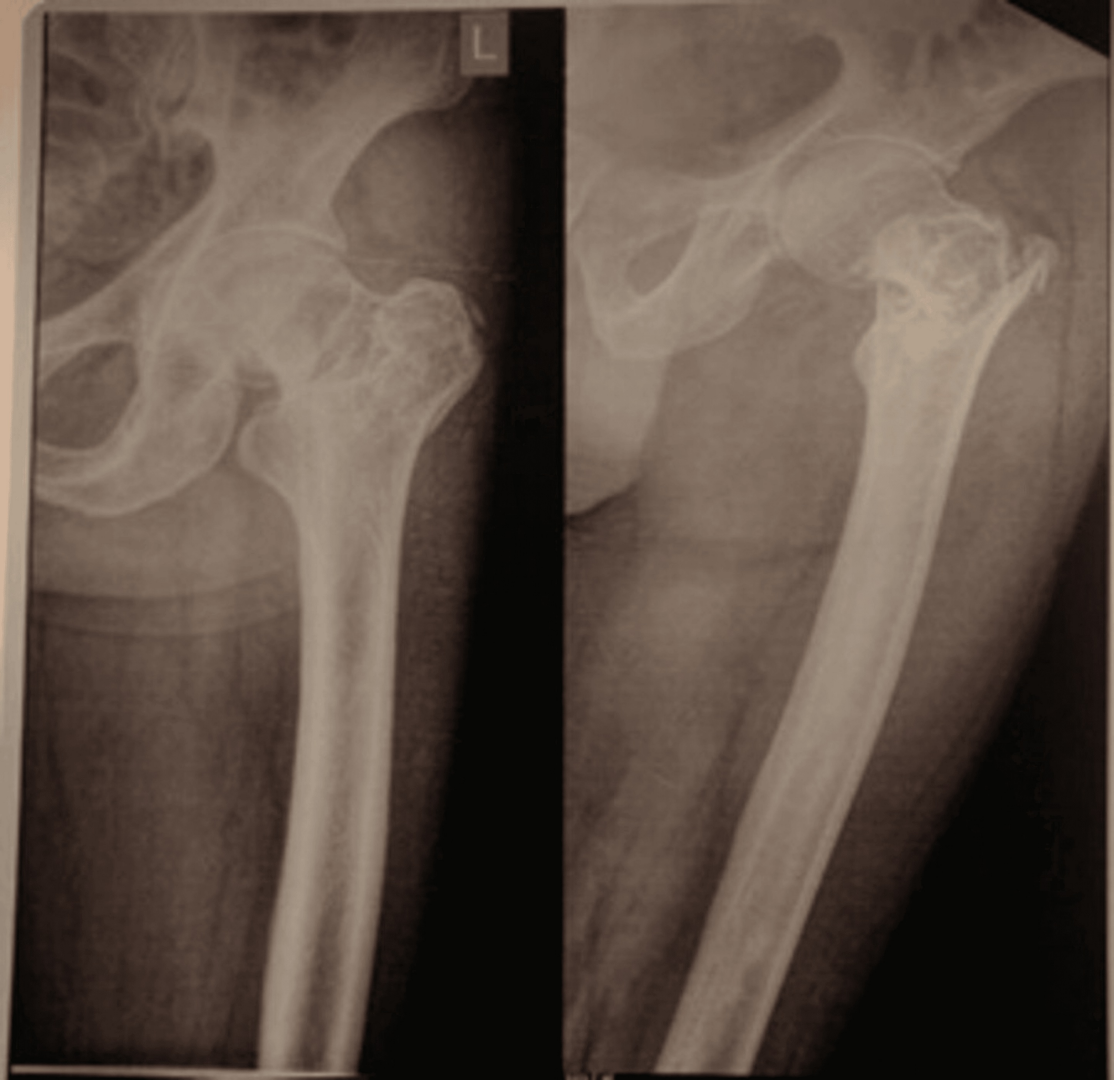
Preoperative radiograph of an adult patient showing left hip intertrochanteric fracture These are anteroposterior (AP) and lateral projections of the preoperative radiograph of the left hip joint and proximal femur of a patient included in the study, showing AO/OTA: Association for Osteosynthesis/Orthopaedic Trauma Association (AO/OTA) type 31A1 intertrochanteric fracture.
